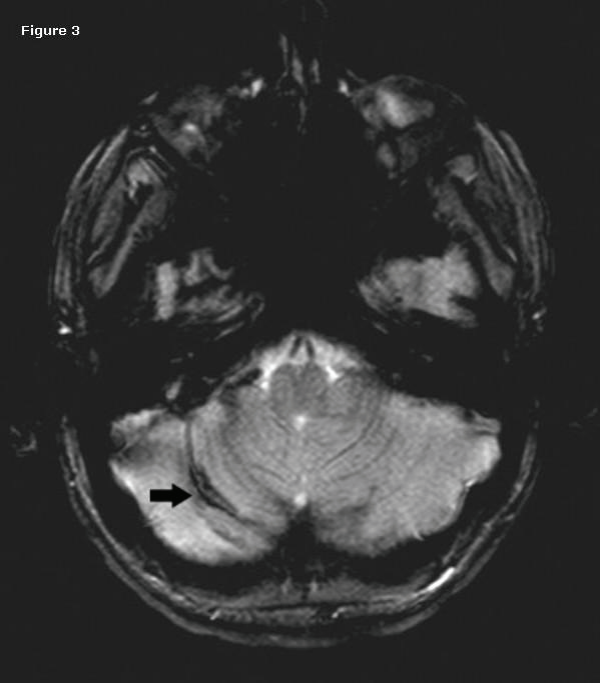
Postoperative first day MRI confirm the hemorrhage. Axial gradient T2 weighted images show a right cerebellar linear hypointensity, compatible with remote cerebellar hemorrhage.
